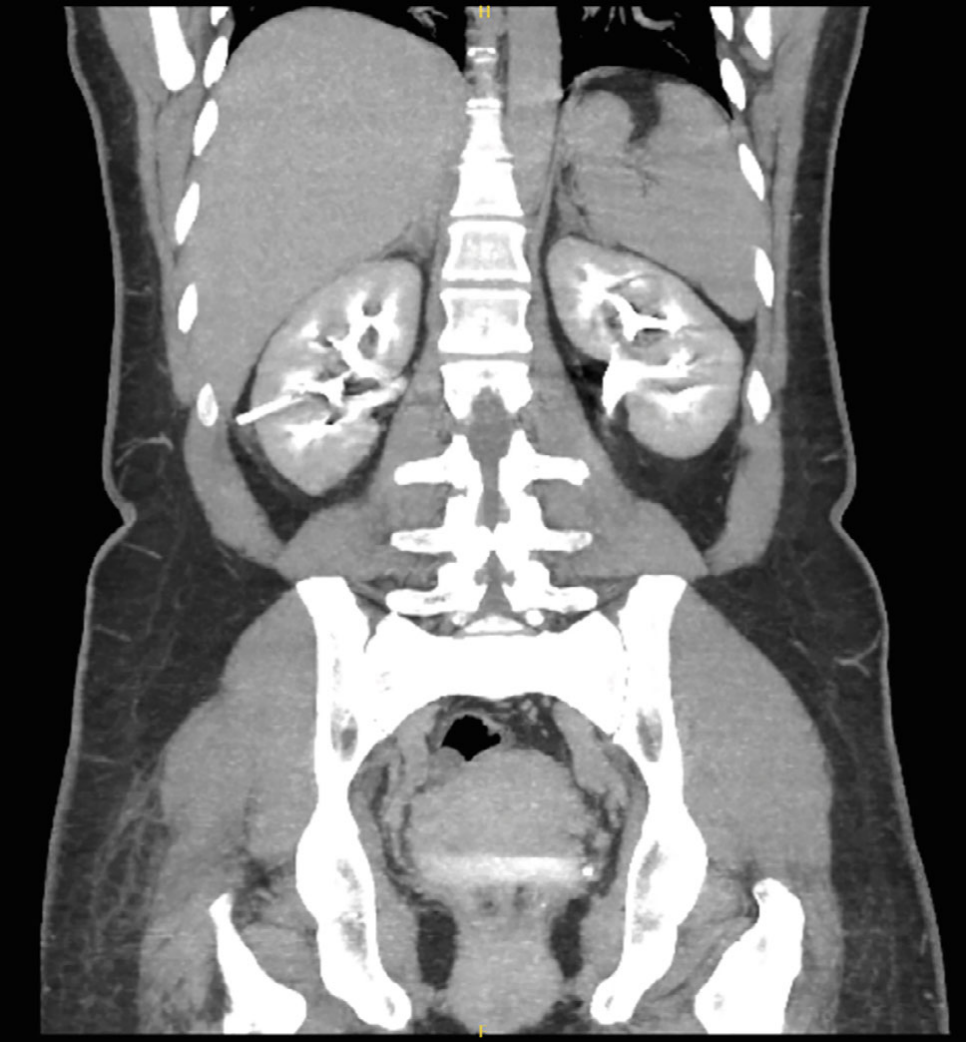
CT urography showing no contrast extravasation, urinary leak, or vascular injury.
